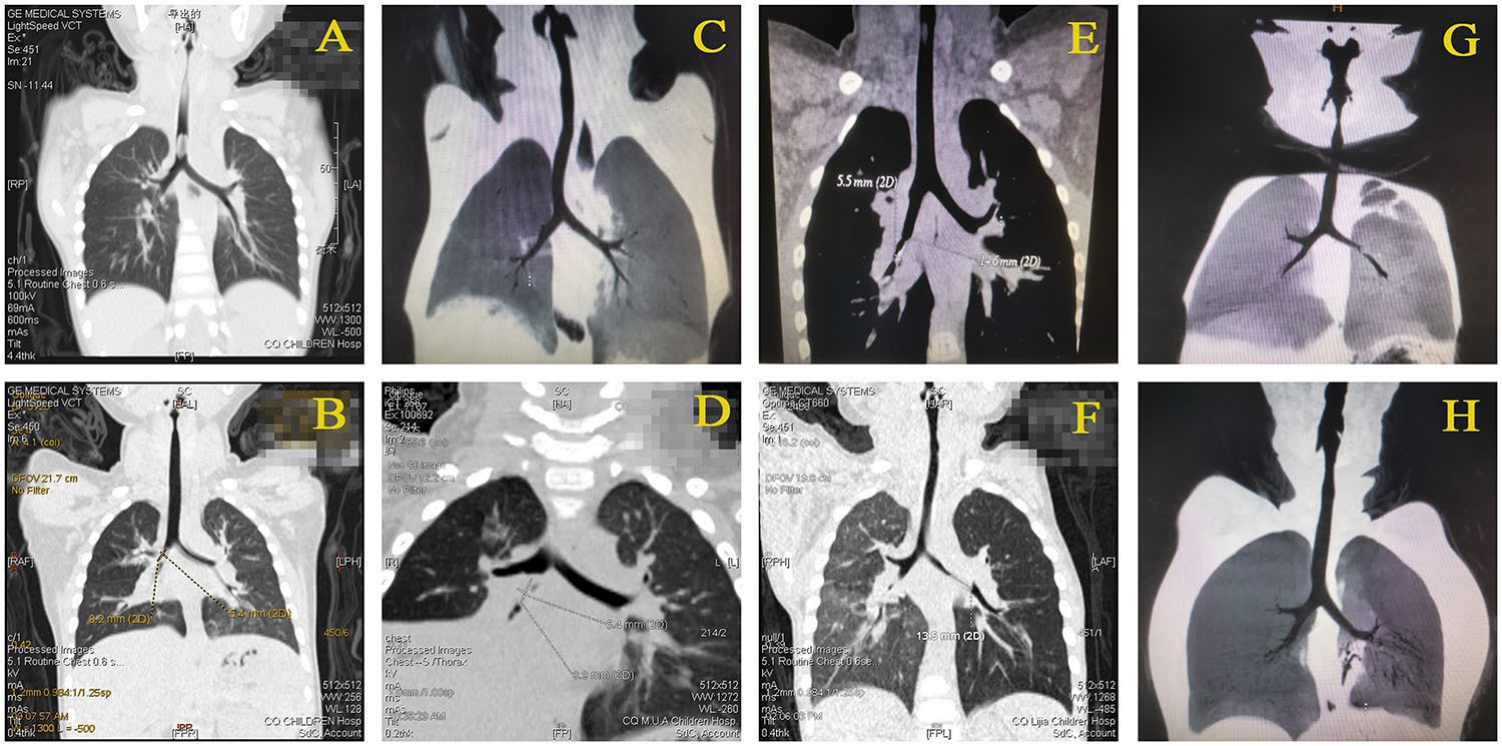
CT images of foreign bodies. A trachea; B right main bronchus; C right superior lobar bronchus; D right middle lobar bronchus; E right inferior lobar bronchus; F left main bronchus; G left superior lobar bronchus; H left inferior lobar bronchus.
